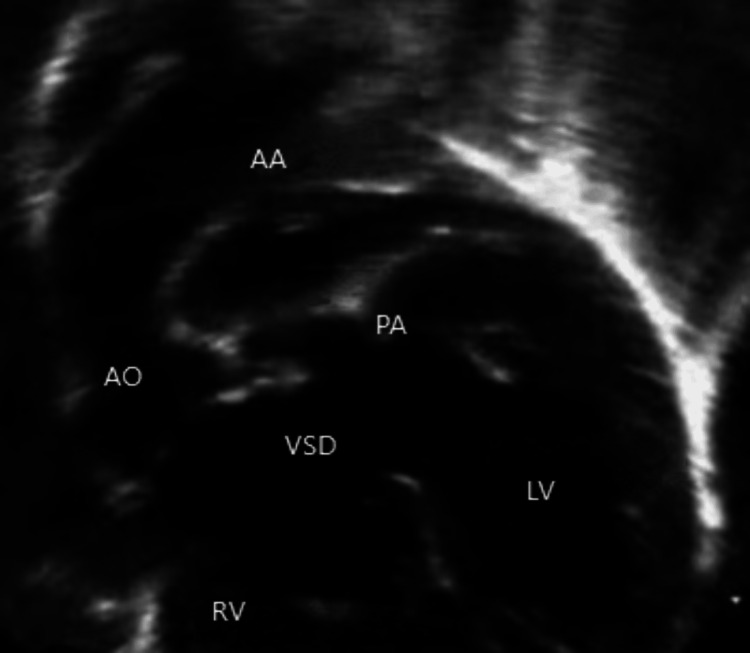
Echocardiogram showing the TOF with PA and PDA from the undersurface of the arch (2D echocardiography view) 2D, two-dimensional; AA, aortic arch; AO, aorta; LV, left ventricle; PA, pulmonary atresia; PDA, patent ductus arteriosus; RV, right ventricle; TOF, tetralogy of Fallot; VSD, ventricular septal defect
